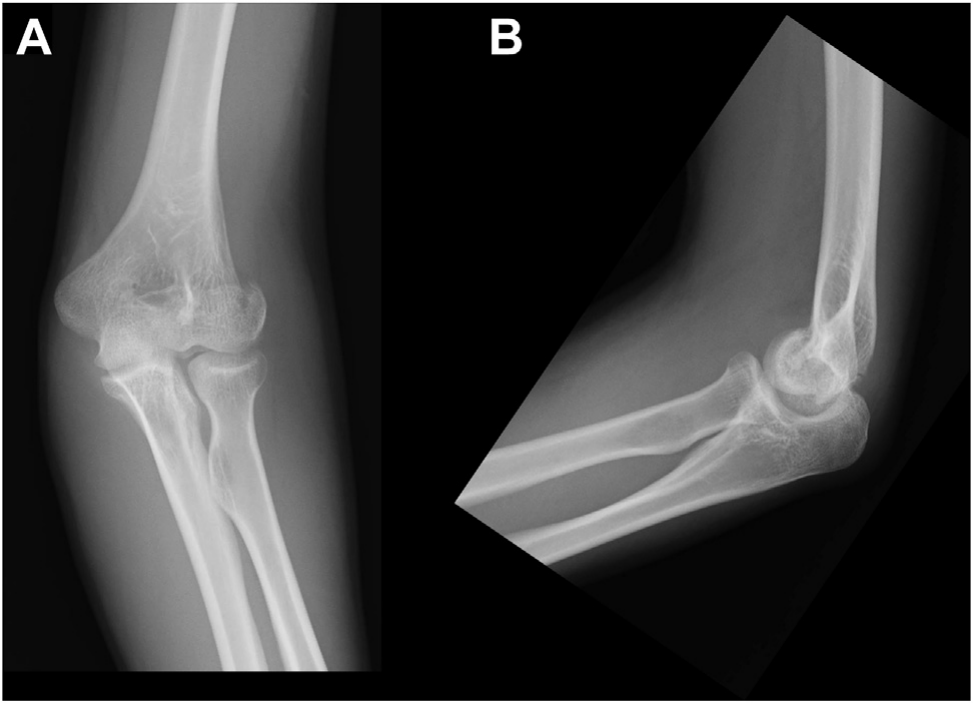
Plain radiograph of the elbow joint at the first visit to our hospital. (A) Anterior-posterior view and (B) lateral view. Coronal shear fracture of the distal humerus is observed. The bone fragment was migrated to the proximal side.
